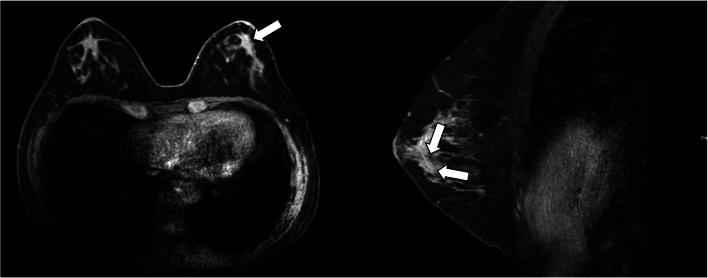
MRI (T2-weighted image) showed a clustered ring enhancement (arrow) in the left lower outer region of the left breast, approximately 9 mm in diameter, which was suspected to be DCIS
